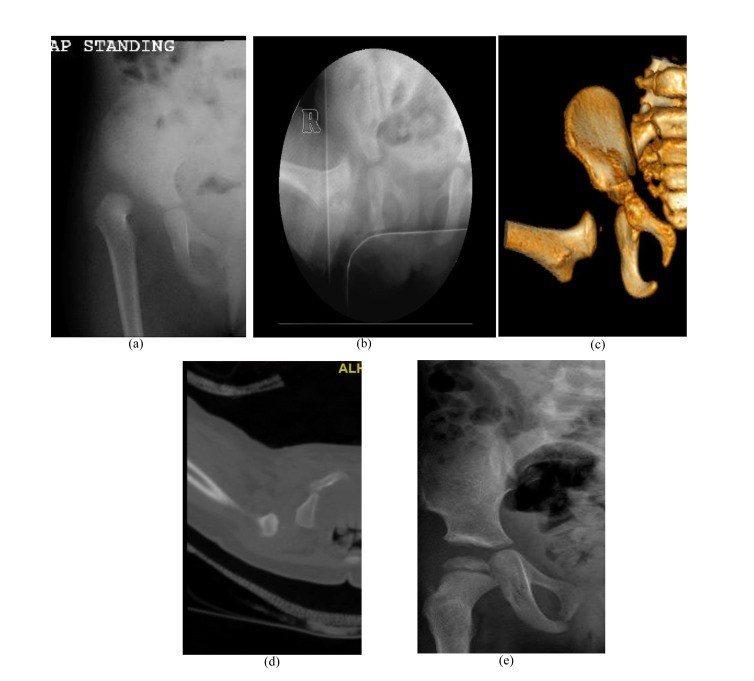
Right hip dislocation in an 18-month old girl. (a) Preoperative radiograph. (b) Intraoperative radiograph after closed reduction and cast application. (c) Frontal 3D CT-reconstructed images. (d) Axial cut showing the posterior dislocation. (e) Follow-up radiograph 22 months after salvage with open reduction and Dega osteotomy.
